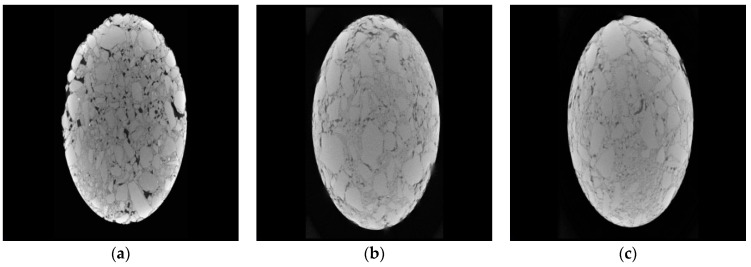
Three-dimensional CT scanning images of R_0_C_0_ (a), R_1_C_0_ (b) and R_1_C_0.09_ (c) mixtures.
